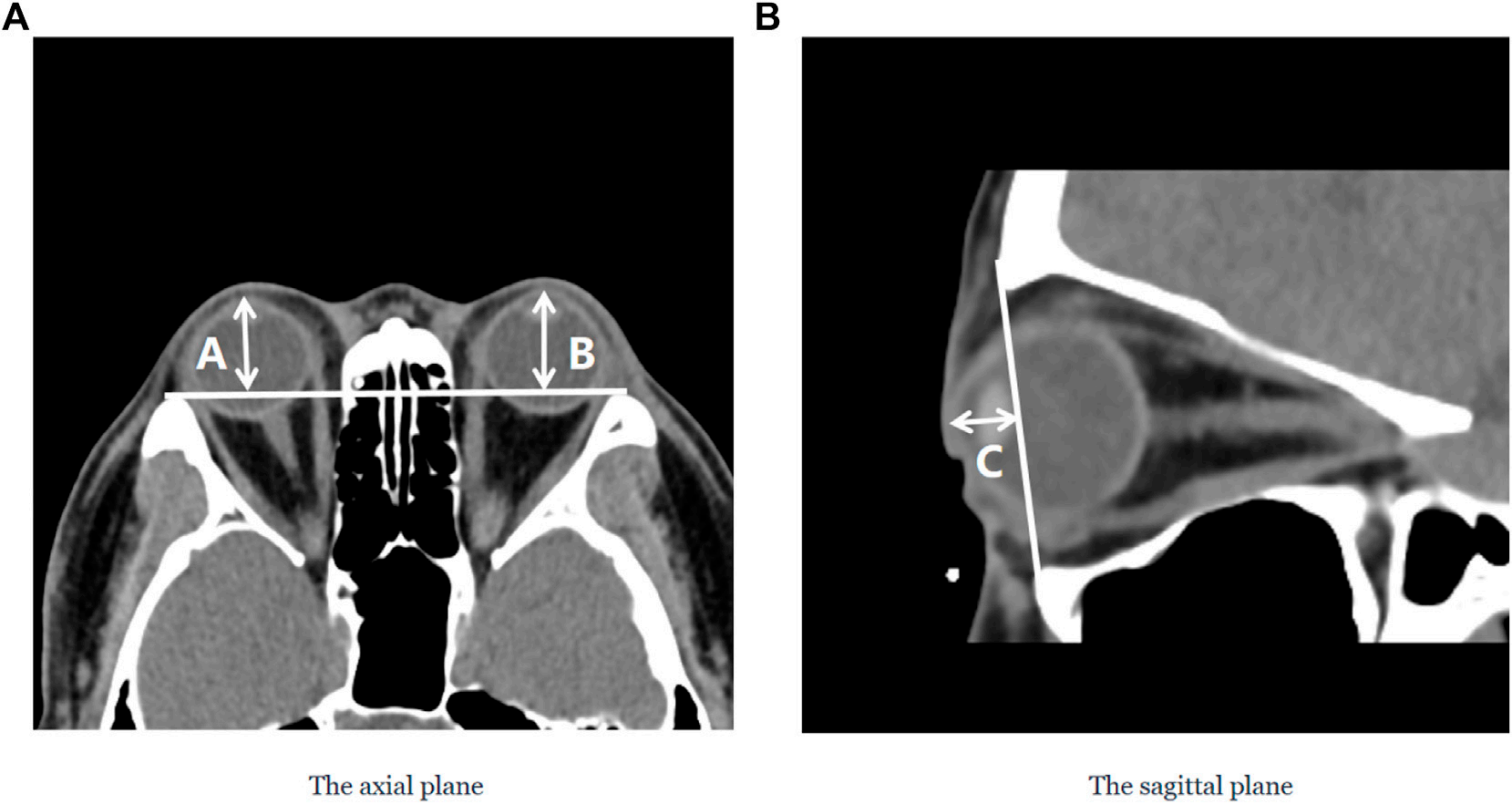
Two commonly used clinical parameters for measuring the prominence of the eye based on CT images. (A) The axial plane of the CT image, (A and B) represent the vertical distance from the apex of the anterior corneal surface to the lateral orbital rim of both eyes, respectively; (B) The sagittal plane of the CT image, C represents the vertical distance from the apex of the anterior corneal surface to the longest line of the upper and lower orbital rims.
